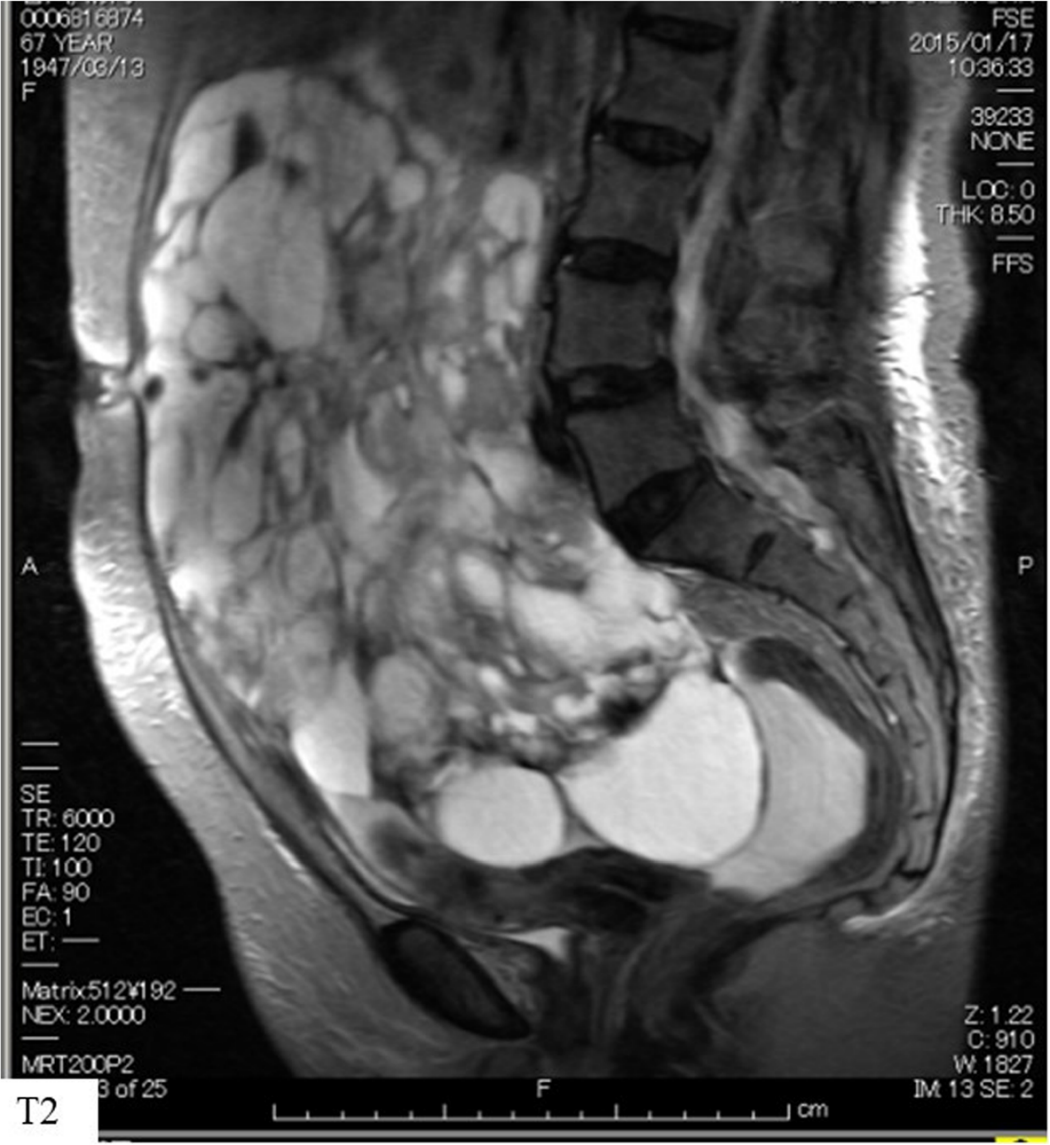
MRI findings. A polycystic pelvic tumor of about 20 cm in size with a solid part was found, and the internal signal on T2 imaging was diverse, with hemorrhaging inside the tumor suspected
